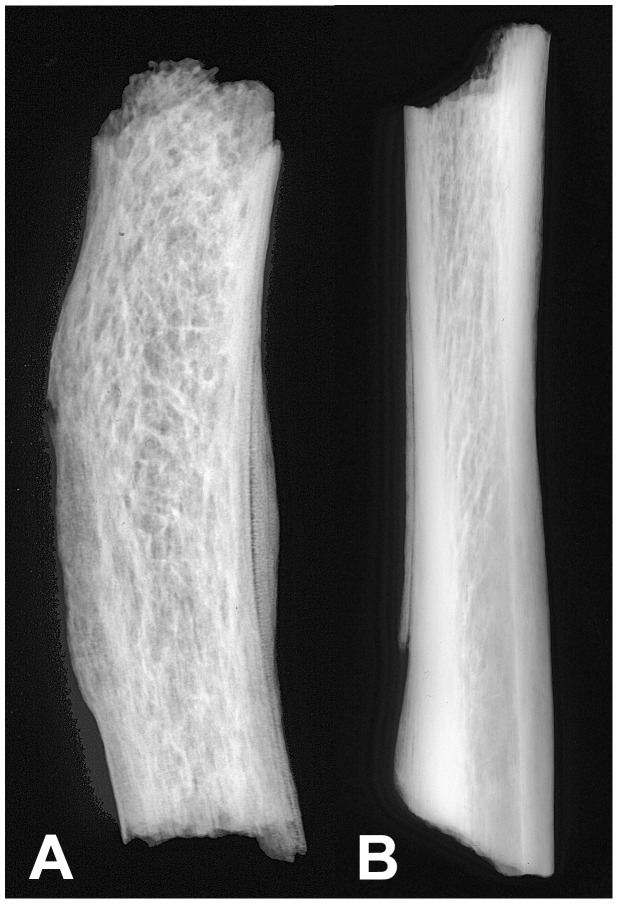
HGO-53– Radiographs. “Appliqué” periostitis on a fragment of rib (A) and a fragment of fibula (B).
